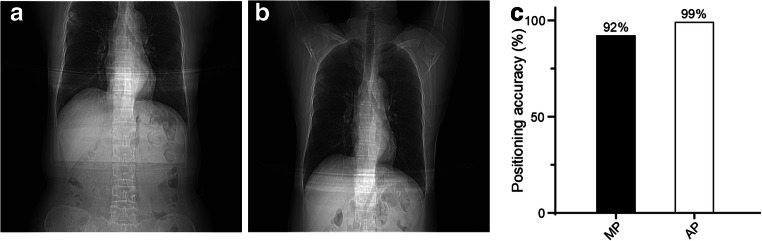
Comparison of positioning accuracy on chest CT topogram. a Inaccurate chest topogram, in which lung field was incompletely displayed. b Accurate chest CT topogram. c Comparison of positioning accuracy for chest topogram acquired by MP and AP (data were presented as *n* (%), where *n* was the number of patients with complete CT scout image; *n* = 127 each)
